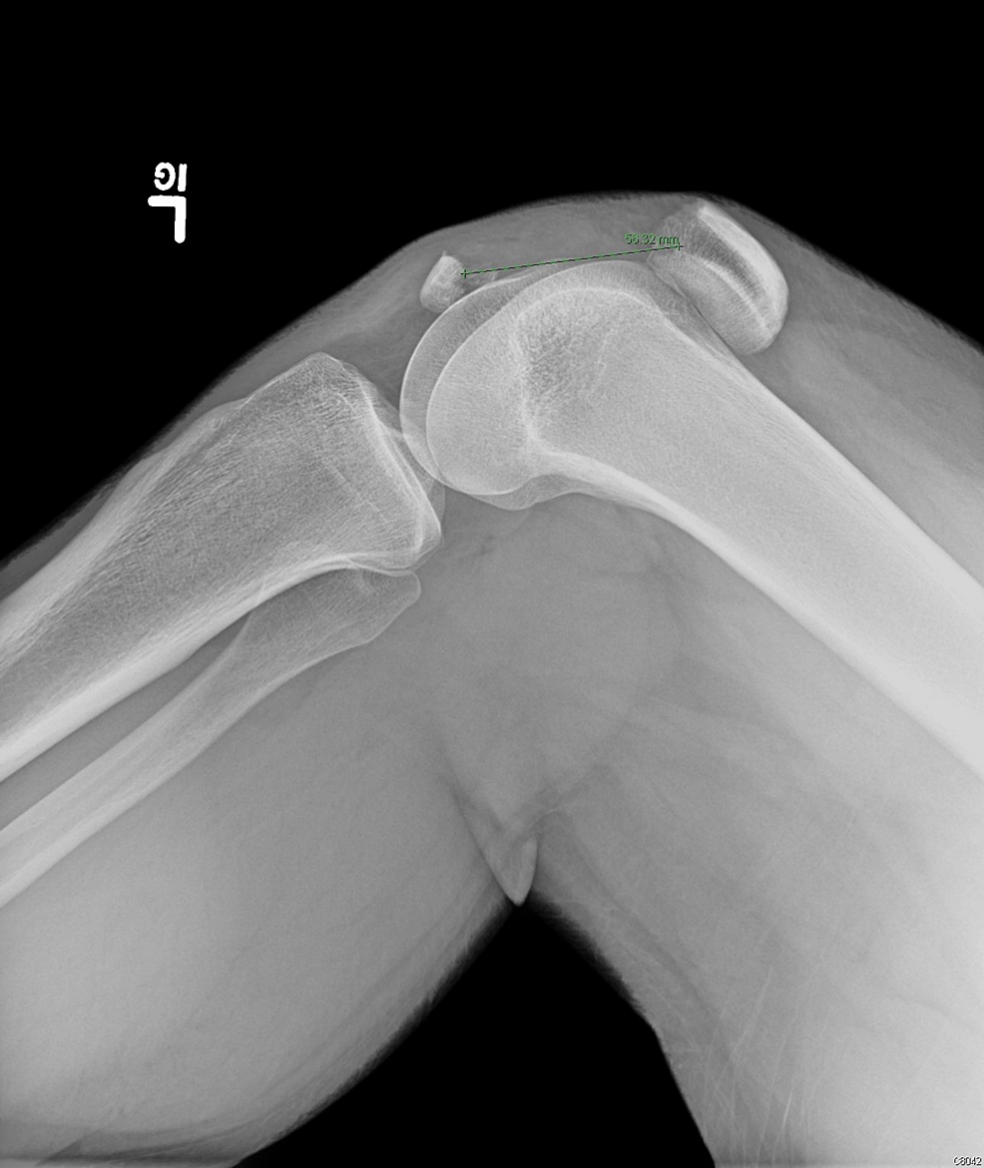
Radiograph of the lateral view of the left knee demonstrating patellar fracture with 56 mm of distraction of fracture fragments
